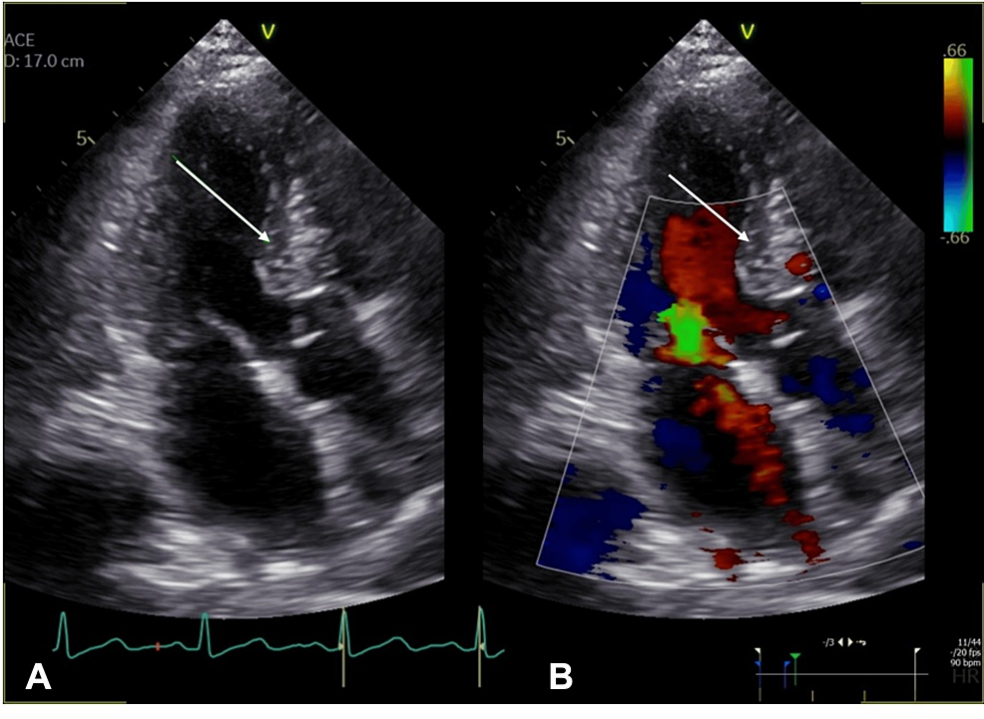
2D transthoracic echocardiogram (TTE) Apical three-chamber view without (A) and with (B) color Doppler showing a suspected mass (arrow) in the basal anteroseptal wall.
